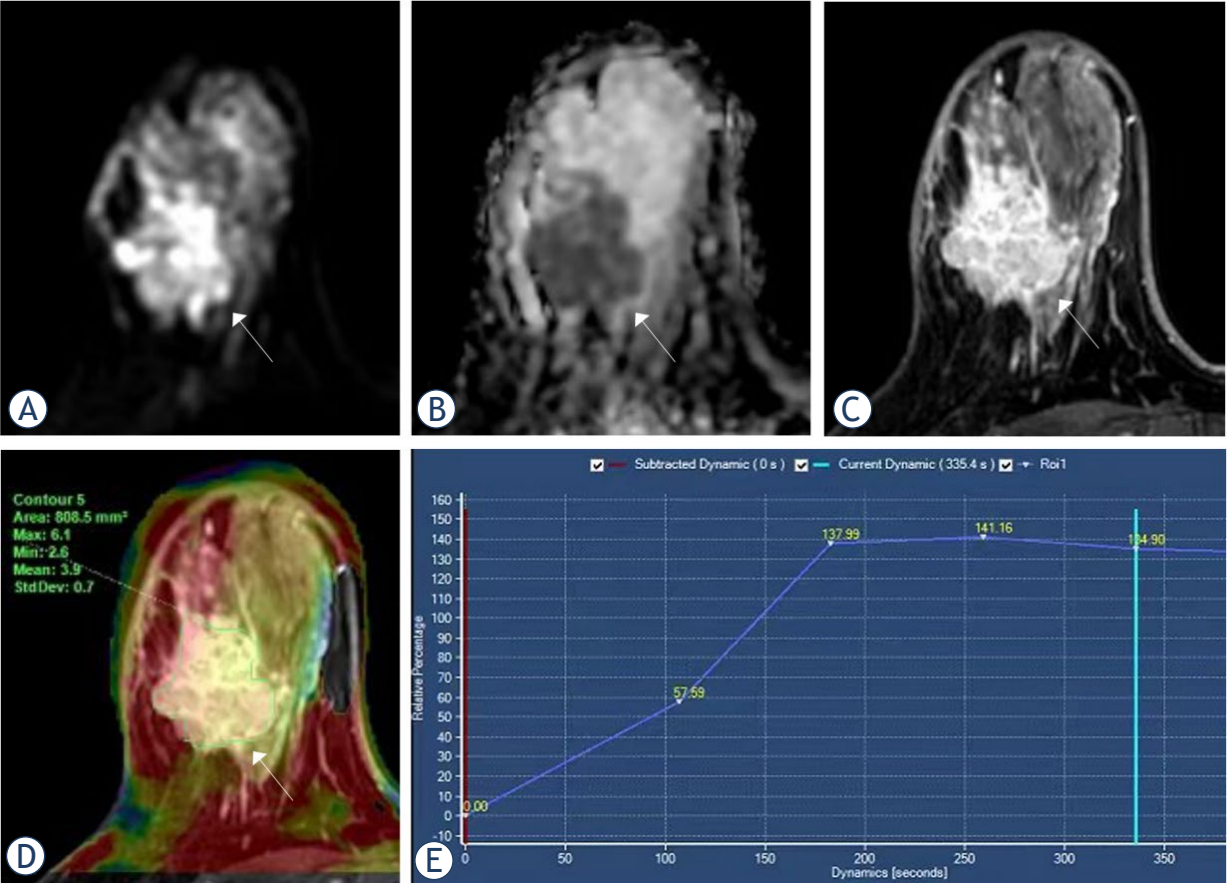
Magnetic resonance imaging (MRI) from a 42-year-old woman with invasive breast carcinoma. (A) DWI = diffusion-weighted imaging (B) apparent diffusion coefficient (ADC) (C) dynamic contrast-enhanced (DCE) (D) The amide proton transfer-weighted imaging (APTWI) pseudo-color image was merged with the DCE images and the APT value was 3.9%. (E) The type of time-intensity curves (TIC) was plateau.
